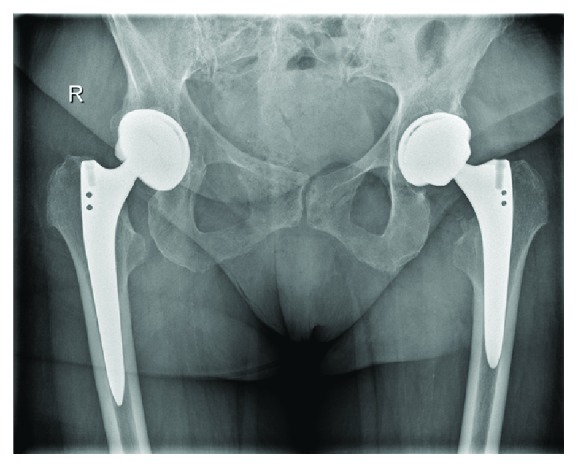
Postoperative X-ray showing left total hip arthroplasty.
